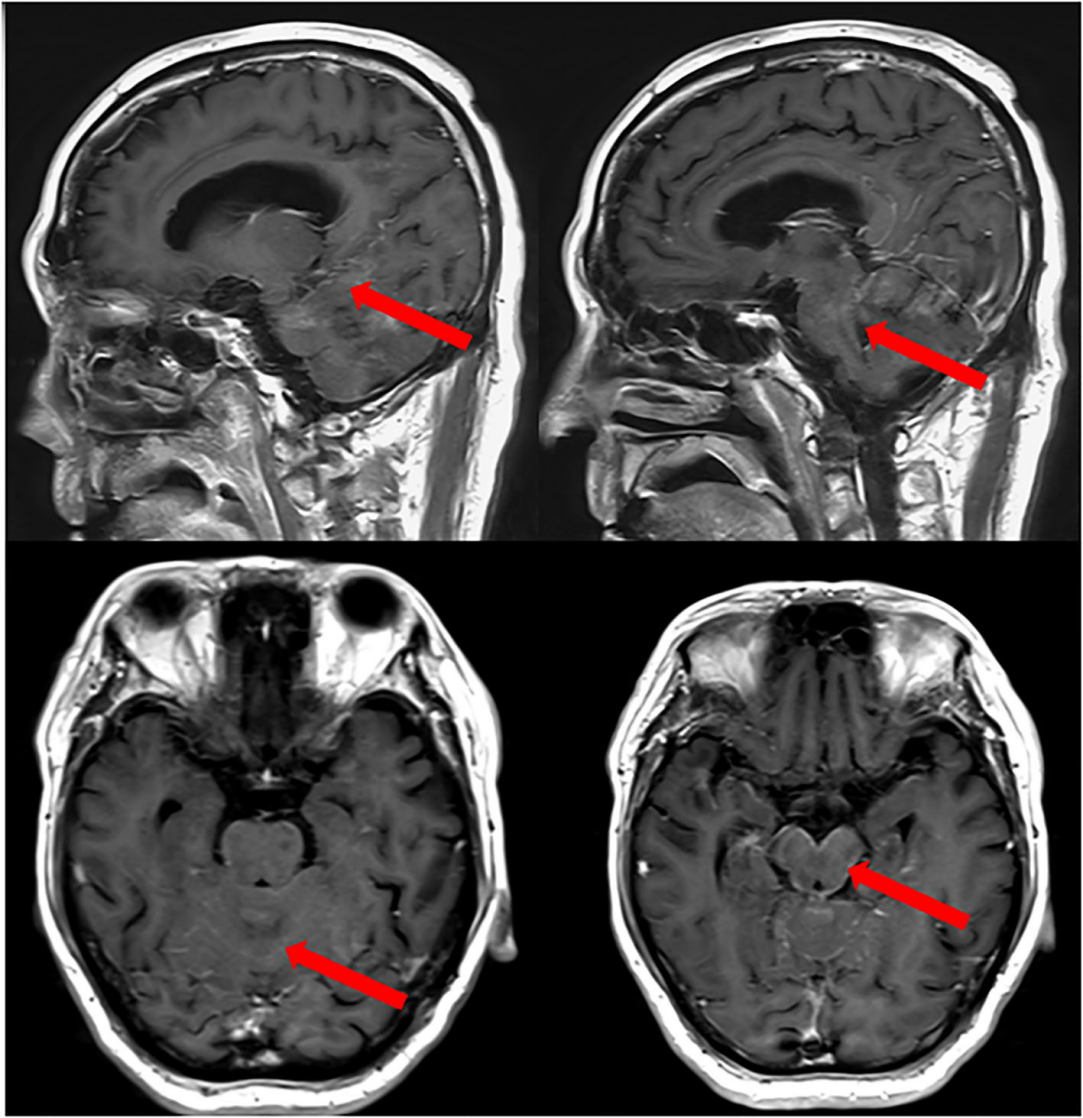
MRI of Case 4 patient with LM−GA. An obvious line−like enhancement was observed on the surface of the cerebellum and pons before the IT combination treatment (red arrows).
